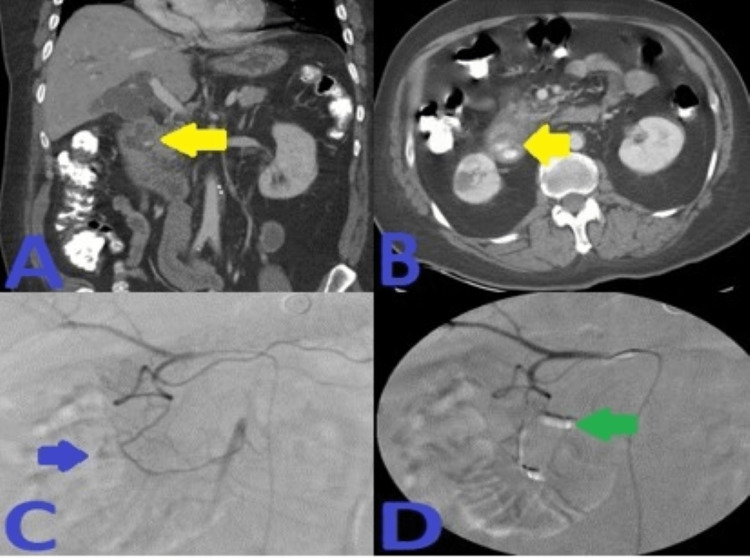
A: CT axial arterial phase demonstrated infiltrative mass with active bleeding in the second part of the duodenum with layering of contrast in the dependent aspect of the duodenum on venous phase as seen on image B. C: Super-selective GDA angiograms using microcatheter system demonstrated active duodenal bleeding from IPDA coming off the GDA with contrast flowing freely and actively from the GDA branches to the duodenum, as seen on CT scan. D: Post-embolization celiac angiogram demonstrated no evidence of active bleeding signs, and complete resolution of the above findings after coils deployment along IPDA in “sandwiching” appropriate positions, beyond the takeoff of gastroepiploic artery, with adequate hepatic arterial flow. CT: computed tomography; IPDA: inferior pancreaticoduodenal artery; GDA: gastroduodenal artery.
